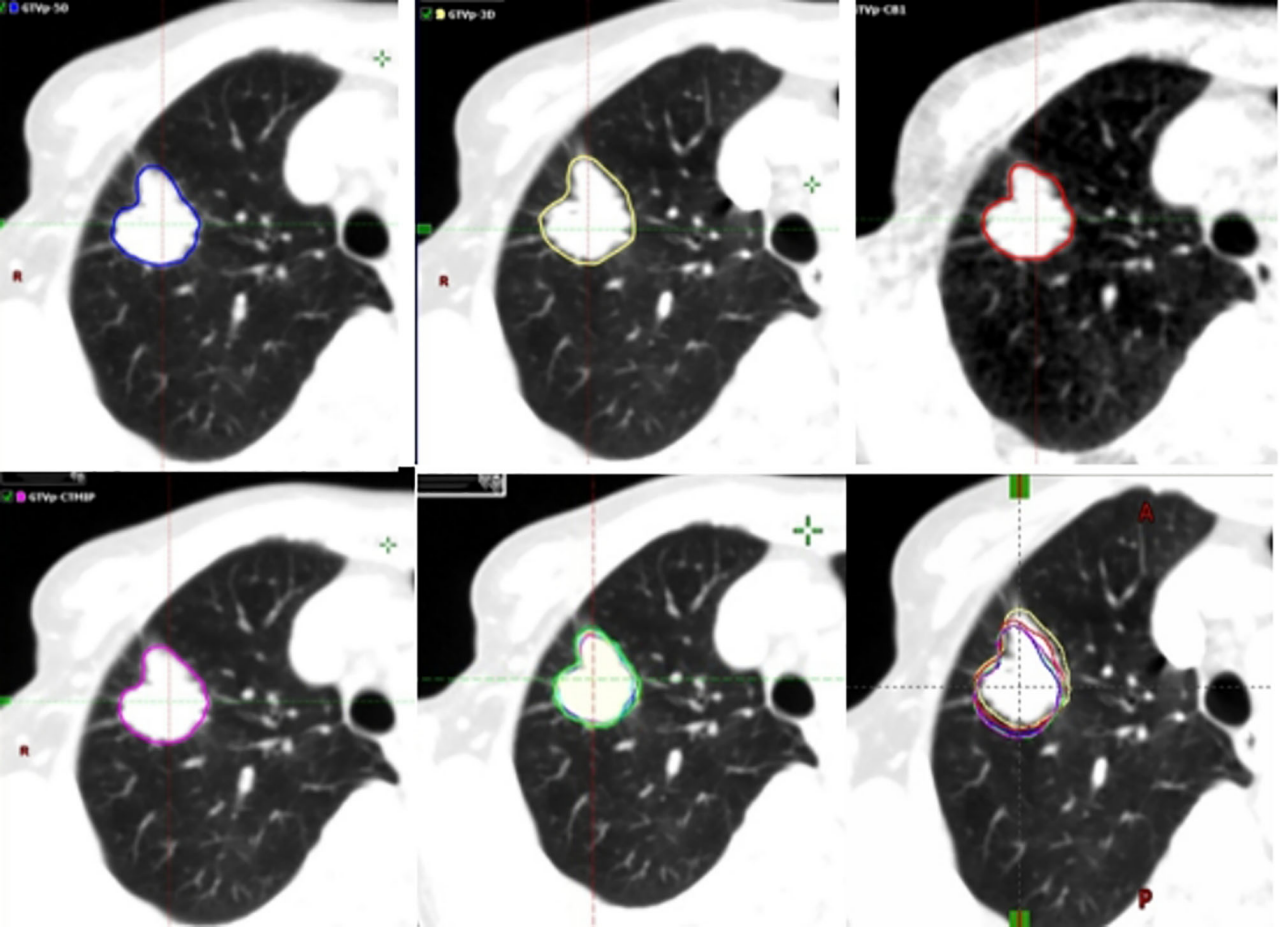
Example (Tumor 132) of the different target volumes. GTV (blue line) contoured based on the end-exhalation of 4DCT, GTV (yellow line) based on 3DCT, GTV (red line) based on CBCT, GTV (magenta line) based on 4DCT MIP, and GTV (green line) derived from ten phases of 4DCT. 3DCT, three-dimensional computed tomography; 4DCT, four-dimensional computed tomography; CBCT, cone-beam computed tomography; GTV, gross tumor volumes; MIP, maximum intensity projection.
